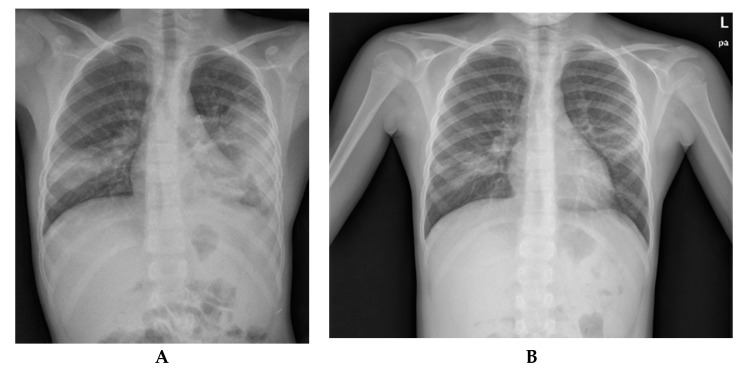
Chest X-ray evolution before allergic bronchopulmonary aspergillosis (ABPA) diagnosis (A) and one month after treatment (B).
